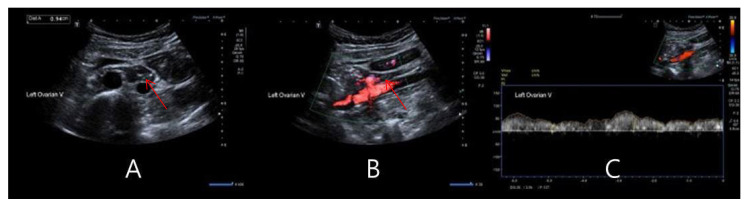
Ovarian vein duplex ultrasonography and measurement of reflux flow and time. (A) Transverse plane of left ovarian vein (red arrow), (B) longitudinal plane with color Doppler of left ovarian vein (red arrow), and (C) reflux time measurement of left ovarian vein.
